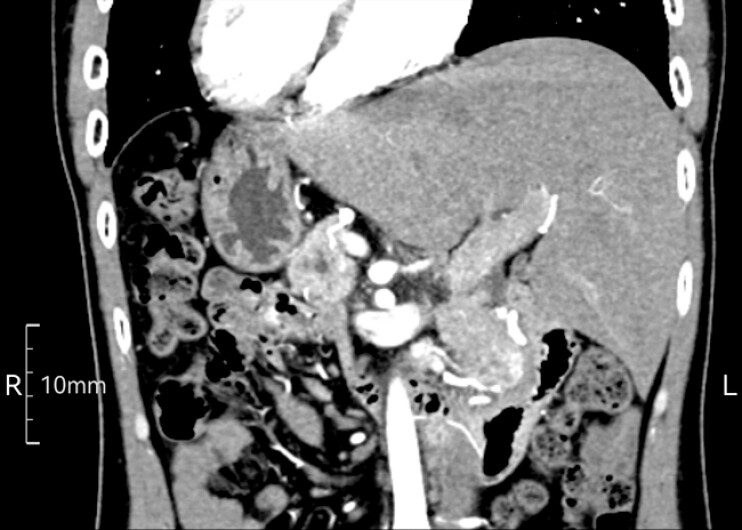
Pre-operative CT revealed heterogeneous enhancement of the pancreatic head, upstream pancreatic duct dilation and situs inversus. CT, computed tomography.
